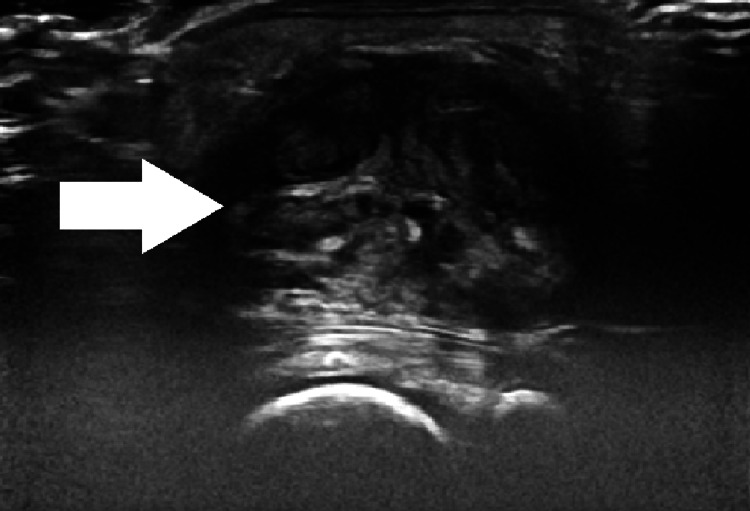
Ultrasound demonstrating a heterogeneous, well-circumscribed swelling in the soft tissue of the right wrist, measuring approximately 41 × 38 mm. The lesion is superficial and localized, with no apparent invasion of adjacent structures.
